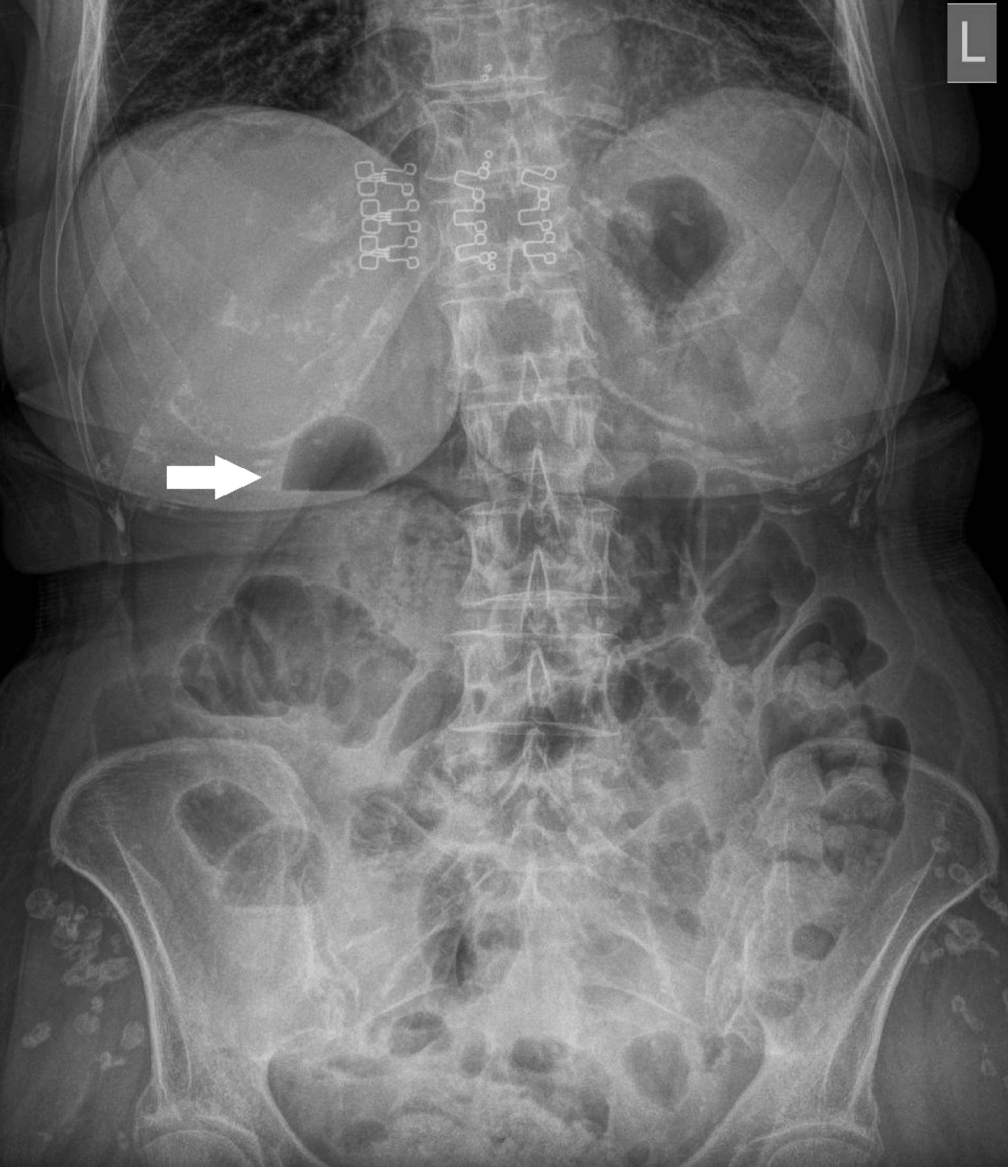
Plain abdominal radiography. A solitary gas-fluid level of the duodenum with luminal distention (white arrow) and several gas-fluid levels of the ascending colon and ileum in the right hemiabdomen, without signs of pneumoperitoneum or pneumoretroperitoneum.
